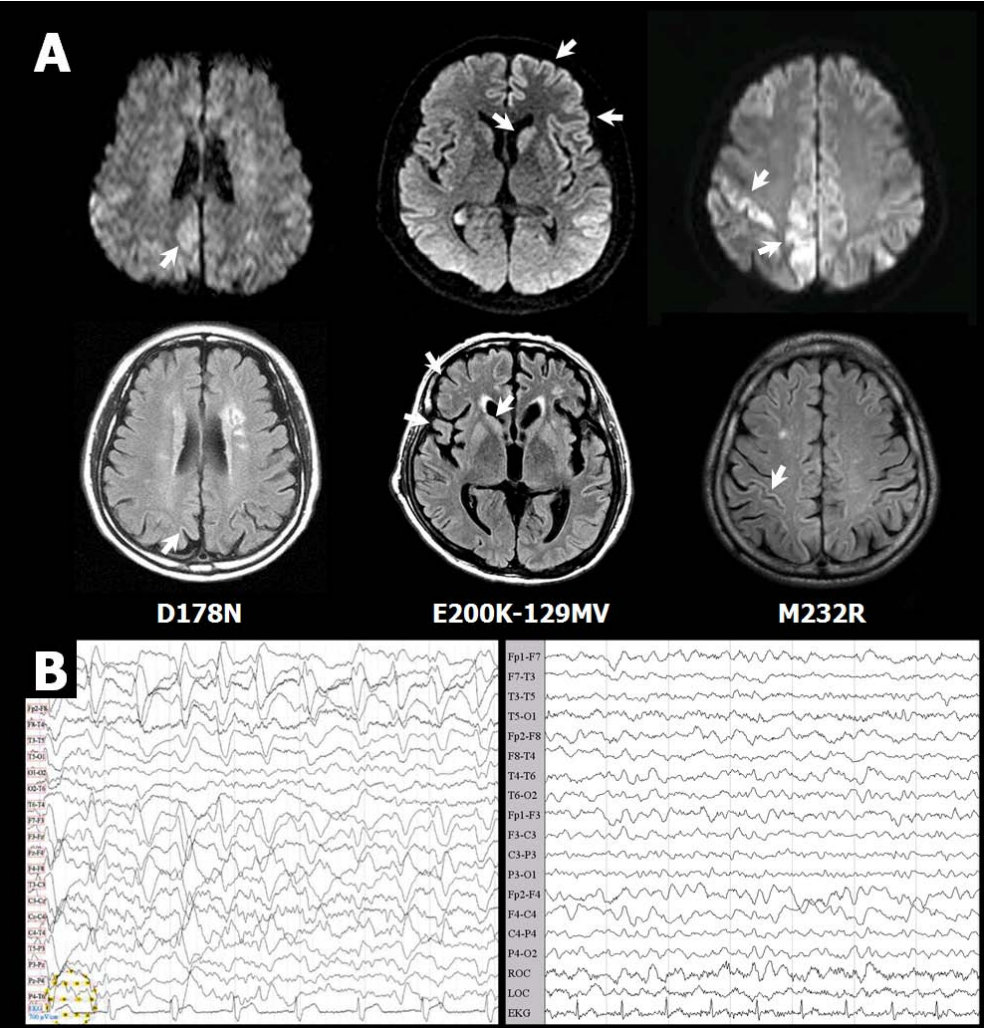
CJD profiles of MRI and EEG from probable CJD patients. (A) Brain MRIs of Cases 1, 2, and 3 with *PRNP *polymorphisms. The two images at the top and bottom left are of Case 1 with the D178N-129M mutation; the two images at center are of Case 2 with the E200K-129MV mutation; and the two images at top and bottom right are of Case 3 with M232R mutation. The top three are DWI images and the bottom three are T2-FLAIR images. The white arrow indicates a lesion with a high signal. (B) EEG of Case 2 (left panel) and Case 3 (right panel). The EEG of Case 1 was normal (data not shown).
